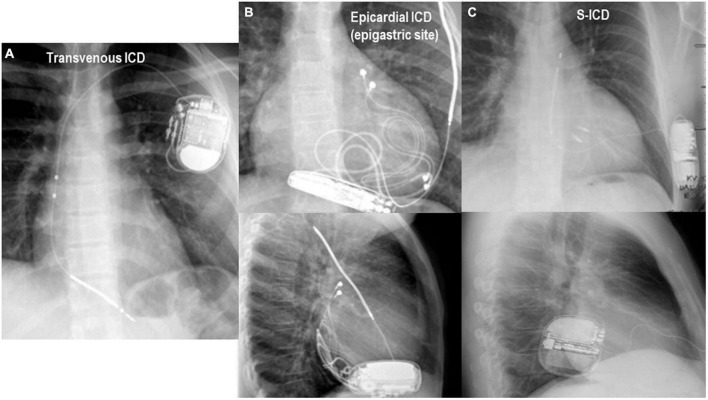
Chest X-ray in anterior-posterior and lateral projections are important for the identification of the type of implantable cardioverter defibrillator (ICD) and for the application of the magnet which should be fixed on top of the ICD can for disabling therapies. (A) Panel shows the typical position of a transvenous ICD immediately below the left clavicula. (B) Panel shows the radiologic antero-posterior and lateral projections in an 8 year-old girl with an epicardial ICD and the can located in the epigastric area. In panel (C), the typical position of the S-ICD system is appreciated.
